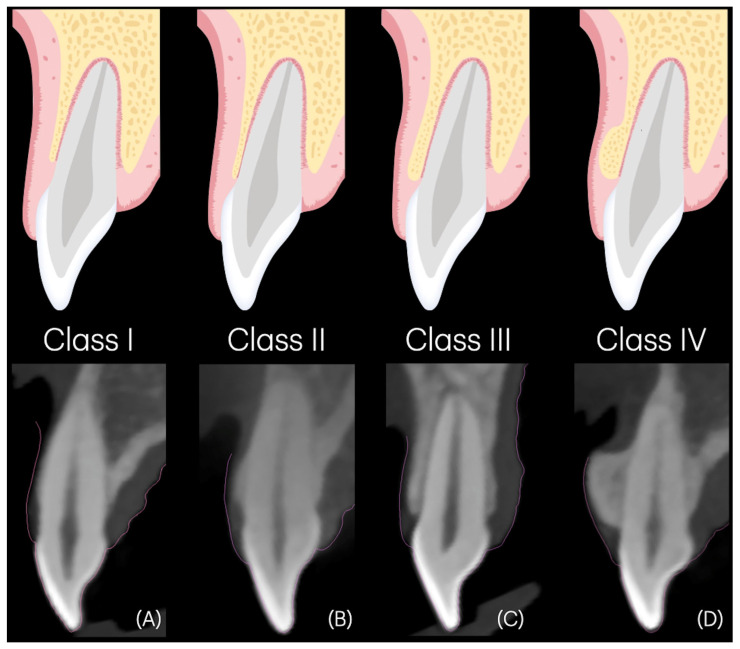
Modified APE classification based on CBCT measurements at the tooth level. (A) Class I: Distance from the cemento-enamel junction (CEJ) to the bone crest (BC) is ≥2 mm, regardless of buccal bone thickness (BT). (B) Class II: CEJ–BC distance < 2 mm, with BT ≤ 1 mm at both BT1 and BT3. (C) Class III: CEJ–BC distance < 2 mm, with BT > 1 mm but ≤2 mm at both BT1 and BT3. (D) Class IV: CEJ–BC distance < 2 mm, with BT > 2 mm or the presence of buccal exostosis.
